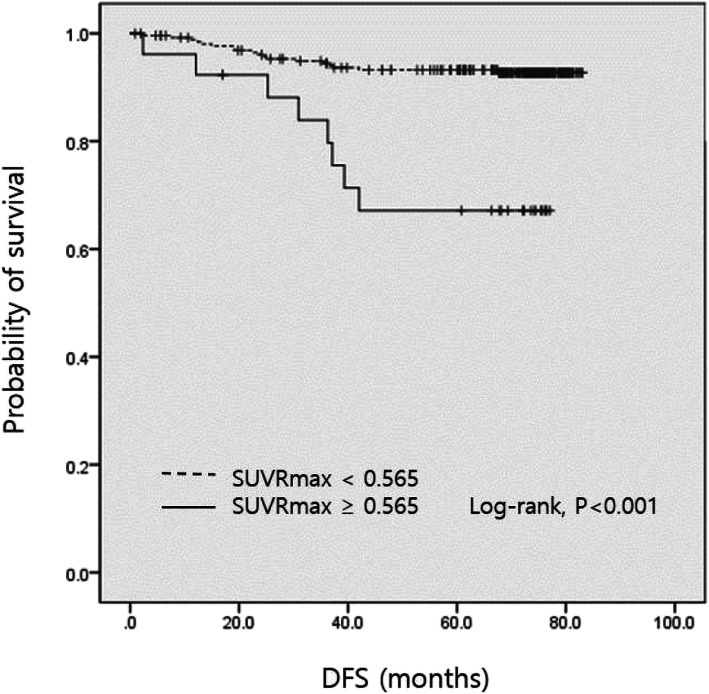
Disease-free survival curves by SUVRmax values. DFS, disease-free survival; SUVRmax, ratio of the maximum standard uptake value
